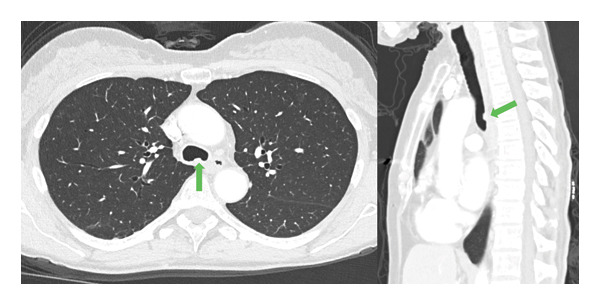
Axial (a) and sagittal (b) chest computed tomography (CT) showing a posterior protrusion (green arrows) in the distal trachea near the carina.
